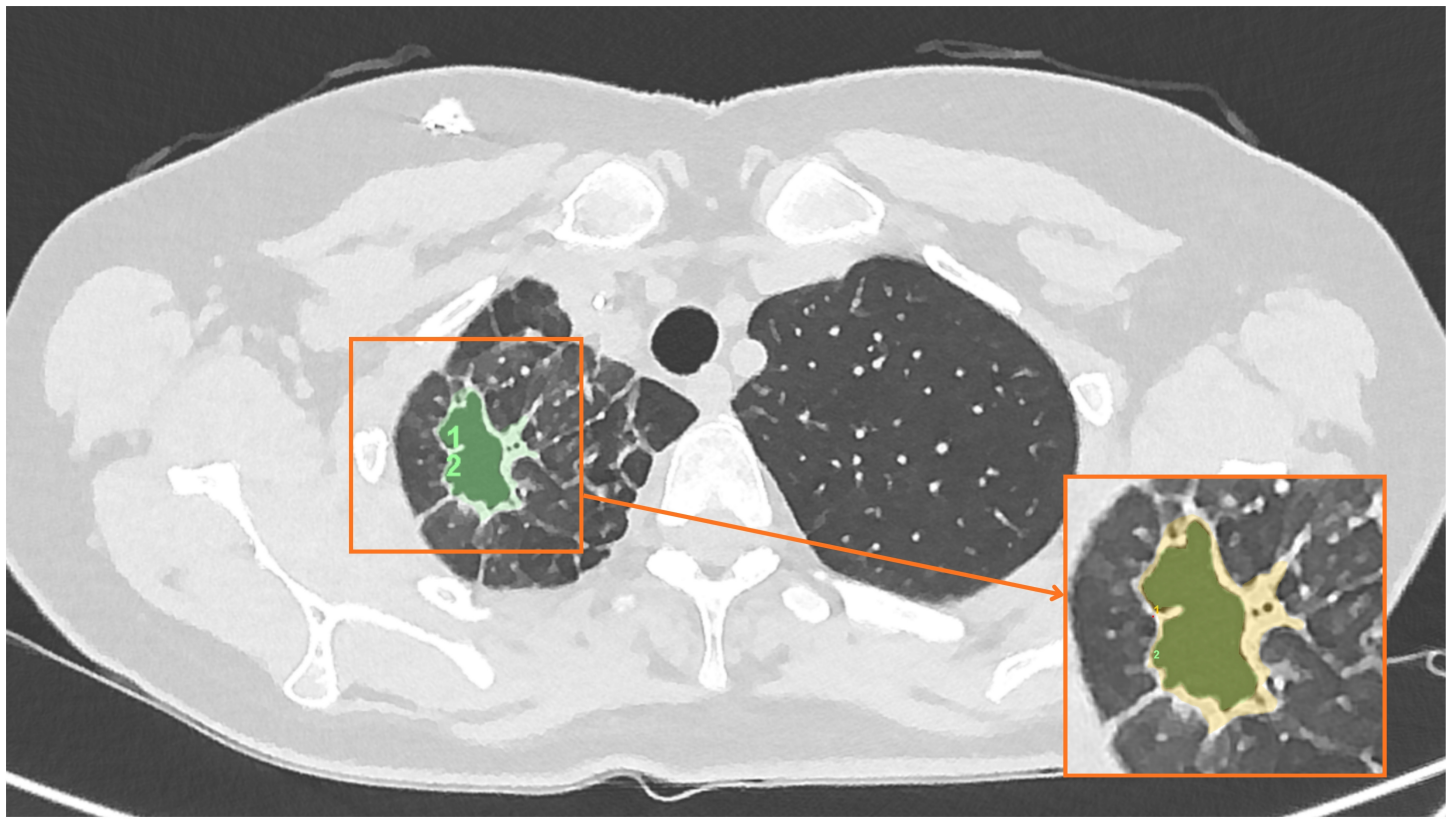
Low-dose CT chest at Day 15 post-treatment initiation demonstrating evolution to a cavitary component within the previously consolidated region, with surrounding parenchymal aeration consistent with interval organization and clearance.
